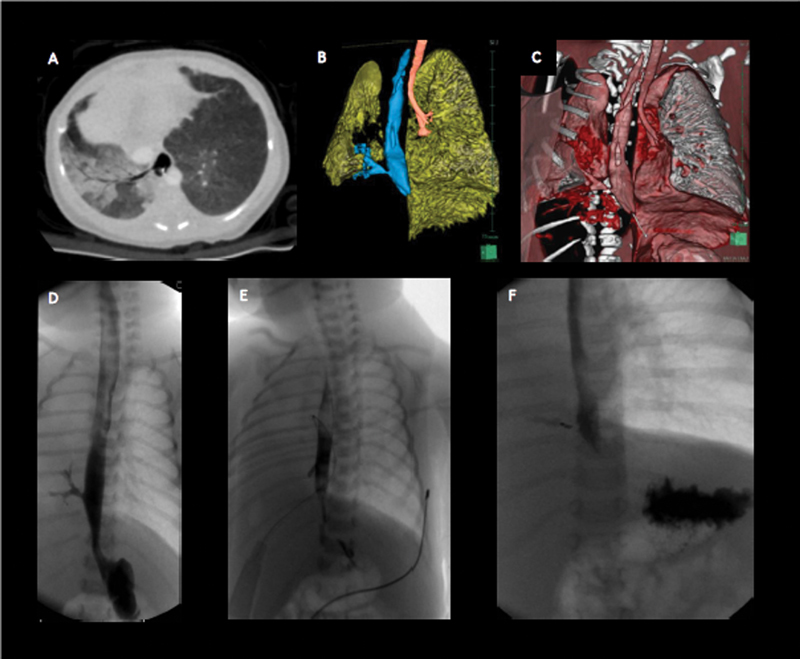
Upper row: CT scan on day 3 of life. (
